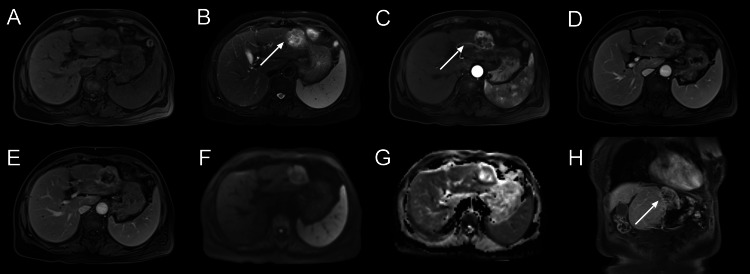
MRI imaging of BAF. (A) T1-weighted image. (B) T2-weighted image. Contrast-enhanced MRI demonstrated markedly heterogeneous enhancement in the (C) arterial phase of the lesion but low signal in the (D) venous and (E) delayed phases. Diffusion and apparent diffusion coefficient (ADC) images (F and G) showed restricted diffusion. (H) Coronal (white arrow). The mass (B) had an obscure border (white arrow). The (C) arterial phase showed that the blood supply came from the left inter-hepatic artery and its branches (white arrow). BAF: Biliary adenofibroma.
